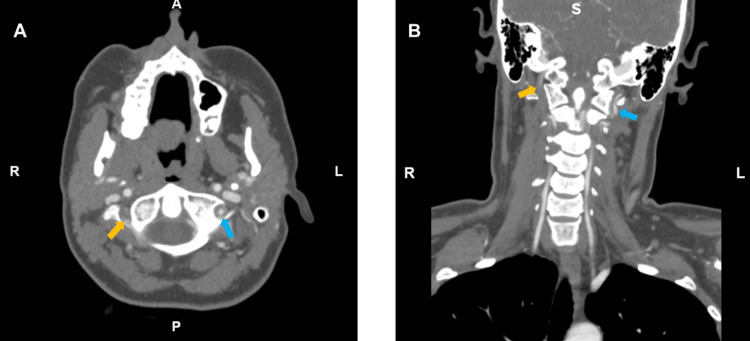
(A) Transverse plane and (B) coronal plane CT angiogram of the neck show severe stenosis of both superior cervical vertebral artery segments starting at the C2 vertebrae level, with thin residual hairline lumens seen at the C1 vertebrae (indicated by light blue and gold arrows). The left vertebral artery dissection ends just prior to the dural margin (indicated by light blue arrow), and the right vertebral artery dissection crosses the dural margin and involves the proximal intradural right vertebral artery (indicated by gold arrow).
